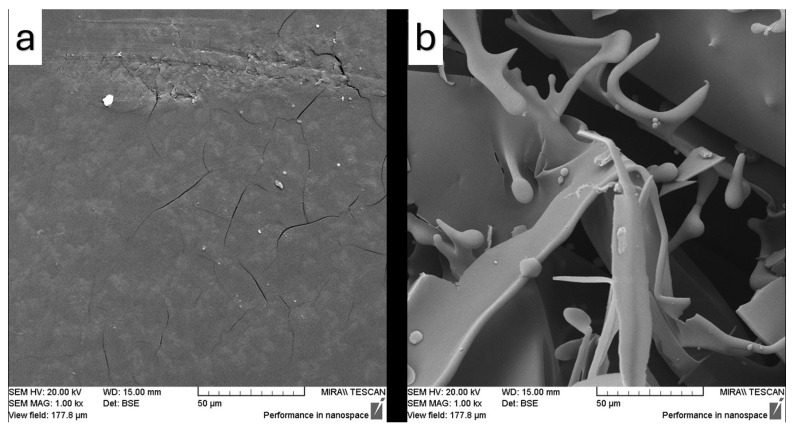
SEM images of (a) ultrasonicated formulation dried using the sample concentrator (SolFenSon3SC), 1000× magnification; and (b) ultrasonicated formulation, which was freeze-dried (SolFenSon3FD), 1000× magnification.
